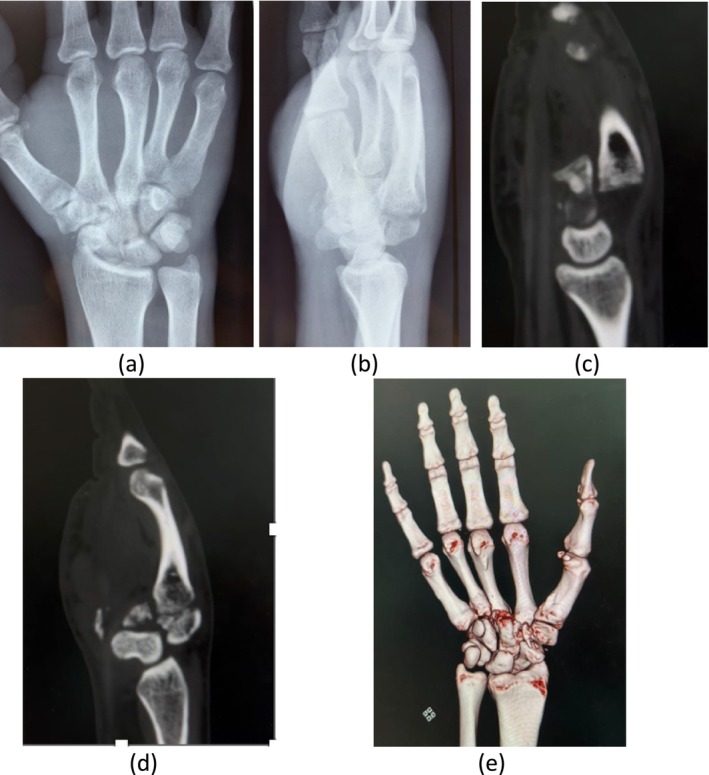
(a) *x*‐ray of hand in AP view, (b) lateral view, (c) CT scan sagittal view of hand showing dorsal displacement of 3rd metacarpal, (d) CT scan sagittal view of hand showing fracture of trapezoid along with dorsal displacement of 2nd metacarpal, (e) CT scan 3D model of hand showing disruption in 2nd and 3rd CMC joint articulation.
